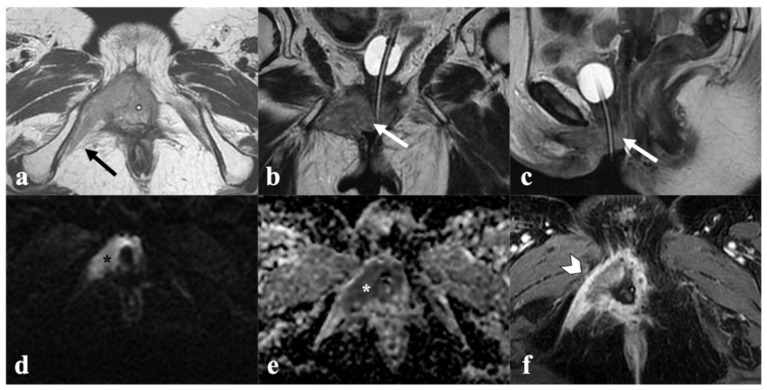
Skin bridge recurrence. A 68-year-old patient previously treated with radical vulvectomy, lymphadenectomy, and RT for locally advanced vulvar carcinoma, presenting 5 years later with a voluminous mass of intermediate T2 signal intensity infiltrating, laterally, the right internal obturator muscle ((a)—black arrow), cranially, the lower third of the vagina ((b)—white arrow), and anteriorly, the urethra ((c)—white arrow). The solid tissue shows diffusion restriction in both DWI ((d)—black asterisk) and ADC map ((e)—white asterisk) and marked enhancement in post-contrast T1-WI ((f)—white arrowhead), consistent with recurrence.
